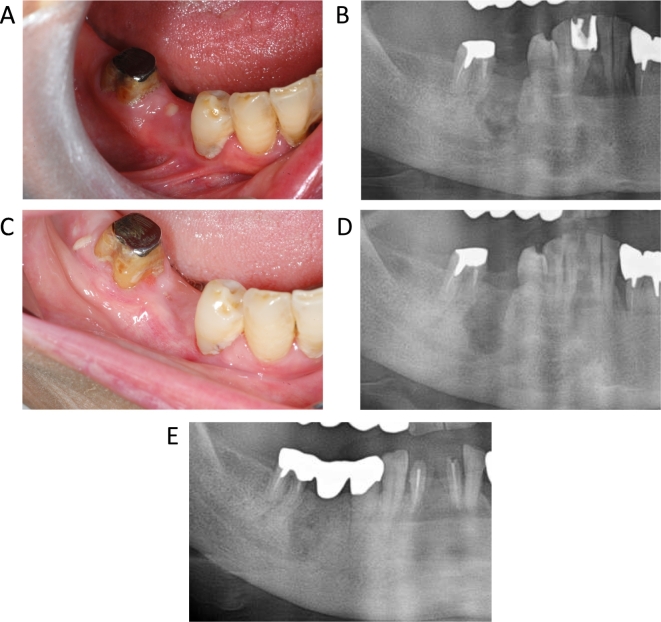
A representative case of a 79-year-old woman diagnosed with stage 2 medication-related osteonecrosis of the jaw (MRONJ) with separation of sequestrum. A: Pus and fistula in gingiva after second premolar extraction. Alveolar bone can be probed through the fistula. B: Separation of sequestrum was observed on panoramic X-ray. C: Complete mucosal wound healing was observed after sequestrectomy. D: Panoramic X-ray after sequestrectomy. E: Two years after surgery, bone regeneration and no recurrence were observed.
